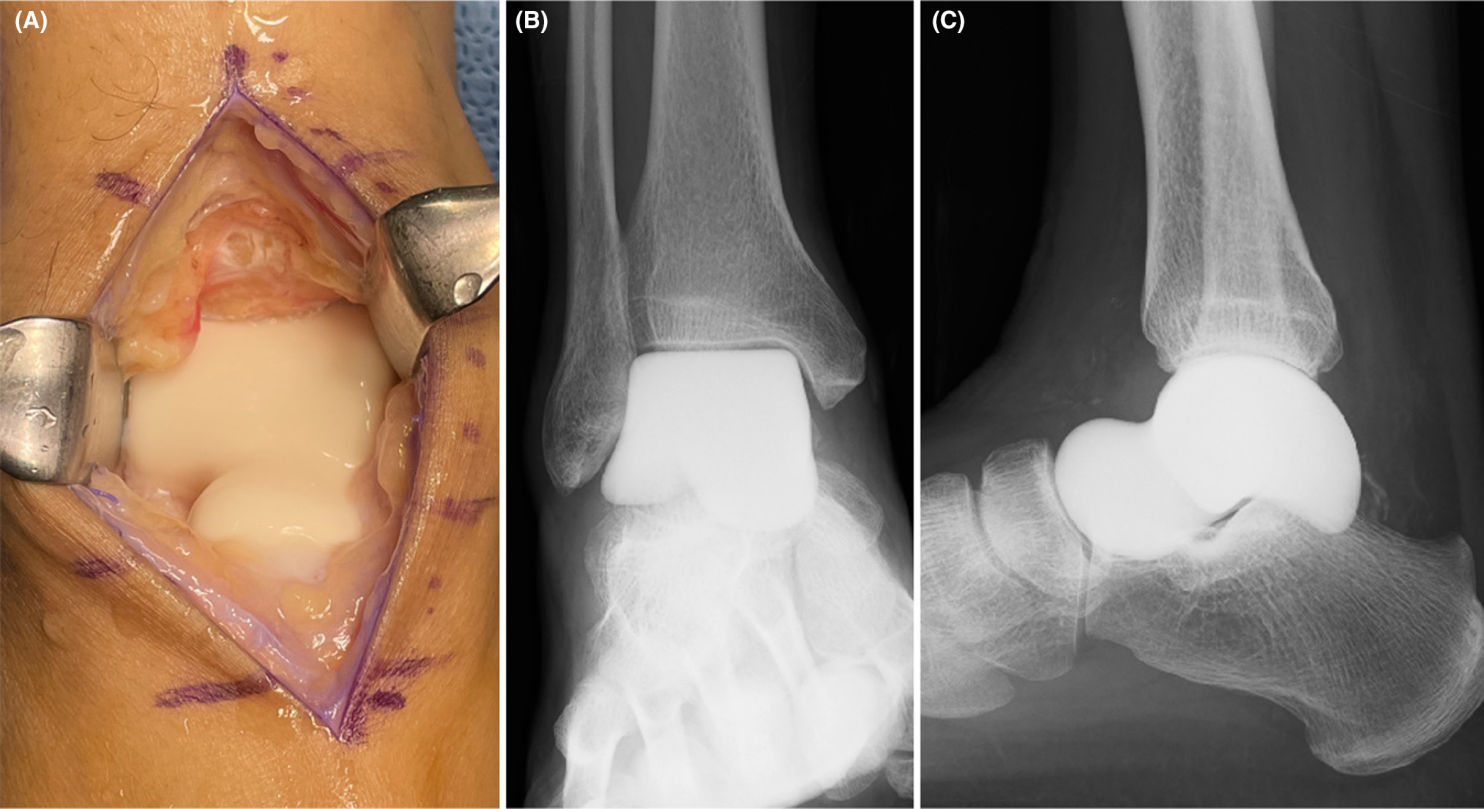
Intraoperative gross findings: after talar replacement (A). Postoperative radiographs of the ankle: frontal (B) and lateral (C).
